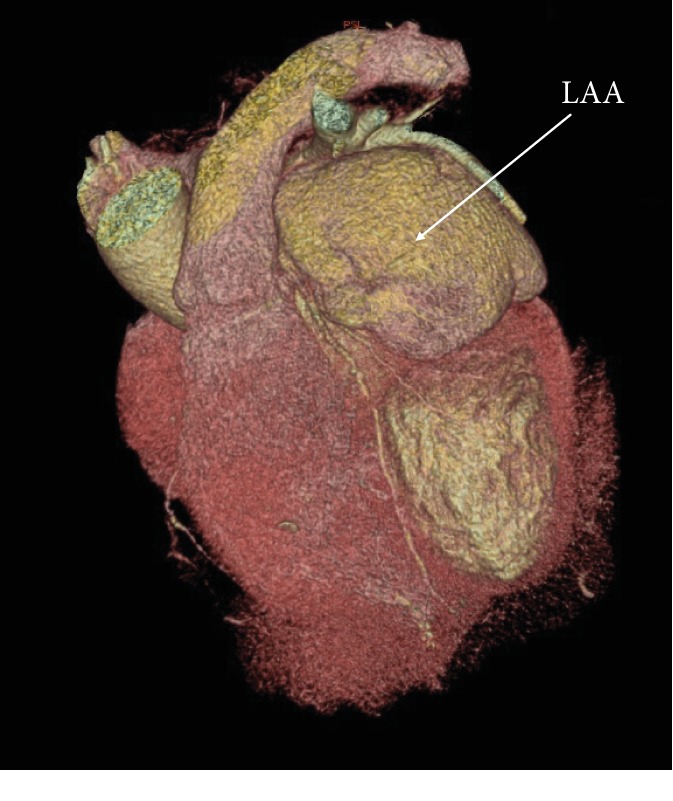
Cardiac CT depicting the LAA. Angiographic “shadow” of the LAA.
